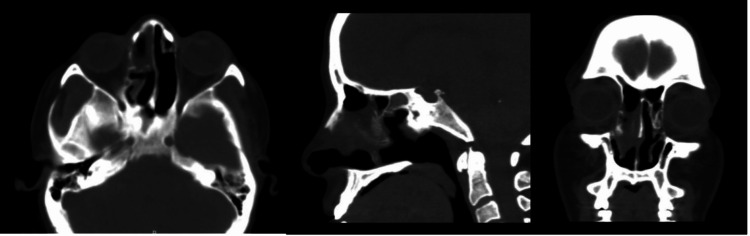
Postoperative CT scan Right frontal sinus osteoma in postoperative view.
